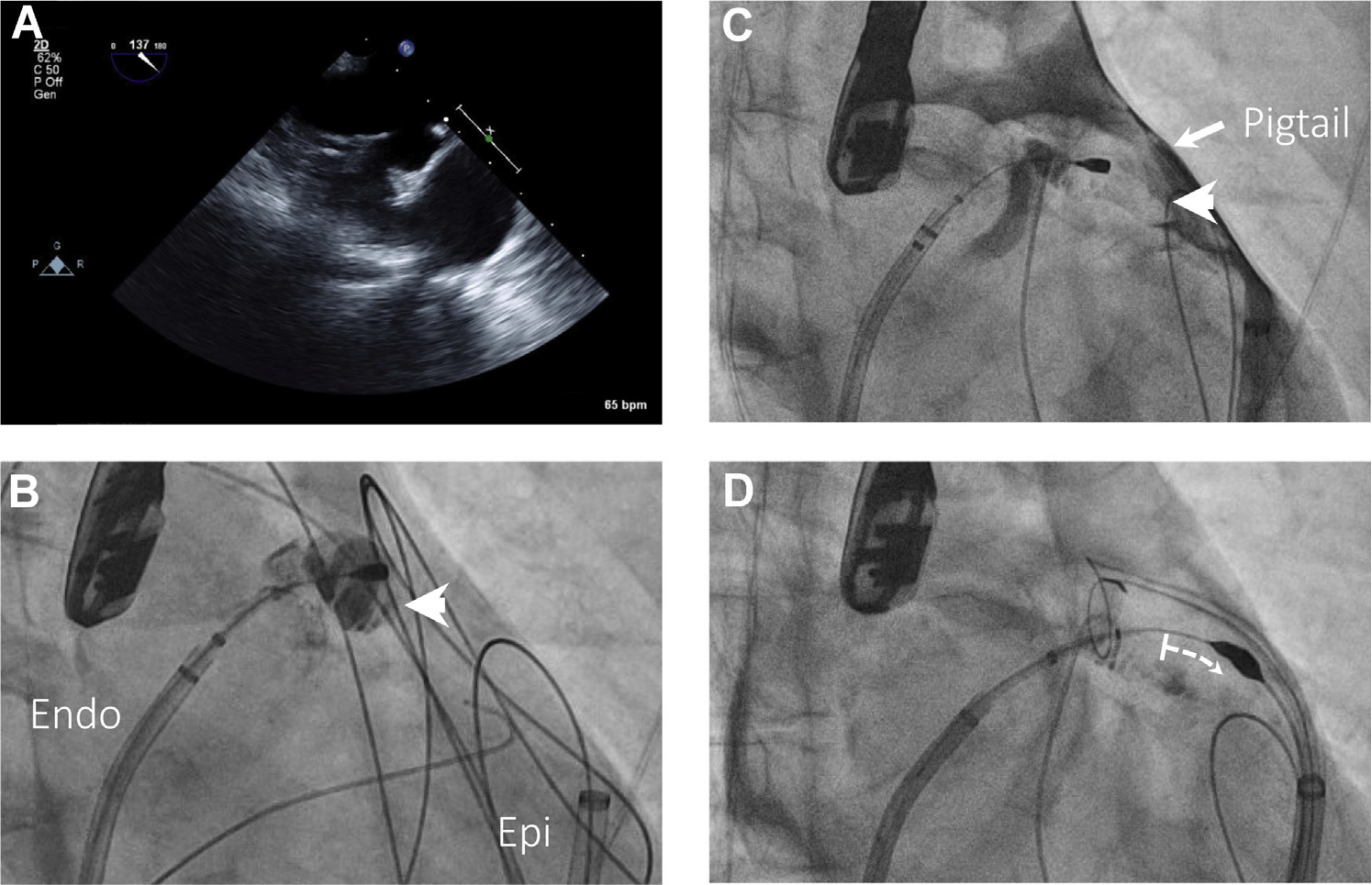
Intraprocedural imaging. A: Intraprocedural transesophageal echocardiography images were suboptimal and visualization of the distal appendage was difficult. B: The endocardial magnet is positioned in the left atrial appendage (LAA). Endocardial injection of contrast through the balloon-tipped catheter falsely suggests that the magnet is in the distal appendage (*white arrowhead*). The endocardial (Endo) and epicardial (Epi) sheaths are labeled. C: Epicardial contrast injection through a pigtail catheter positioned adjacent to the LAA demonstrates that the appendage extends much more anteriorly than was appreciated with endocardial contrast injection (appendage apex labeled with white arrowhead). D: The magnet is advanced into the distal appendage and the structure is ligated. All images were obtained in the same right anterior oblique projection.
